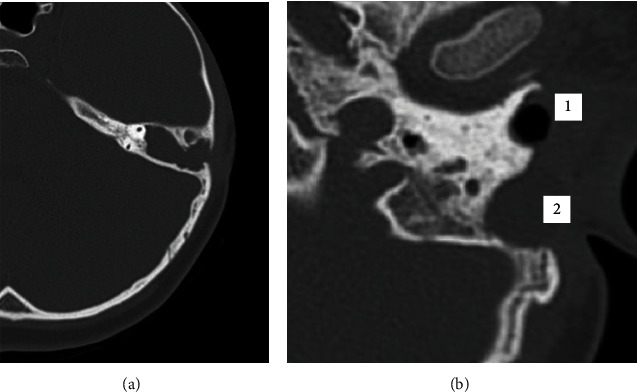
Preoperative computed tomography imaging. (A) Small posterior bone erosions compatible with resorption due to cholesteatoma. (B) Point 1, lower edge of the external auditory canal; point 2, hypointense circular signal in the mastoid compatible with cholesteatoma.
